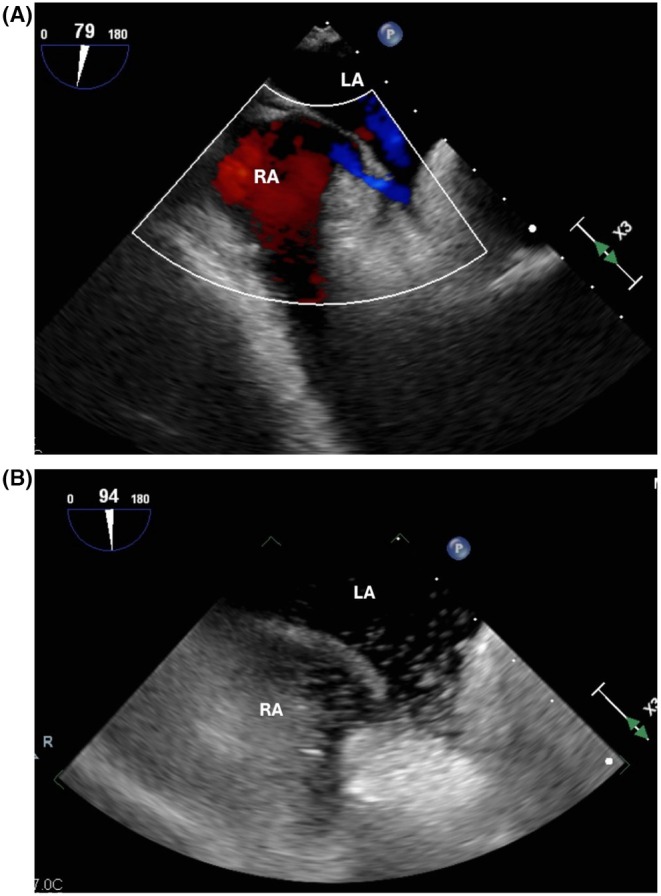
(A) Transesophageal echocardiogram (TEE) with color doppler showing patent foramen ovale (PFO). LA, left atrium; RA, right atrium. (B) TEE with agitated saline confirming right‐to‐left shunting via PFO. LA, left atrium; RA, right atrium.
